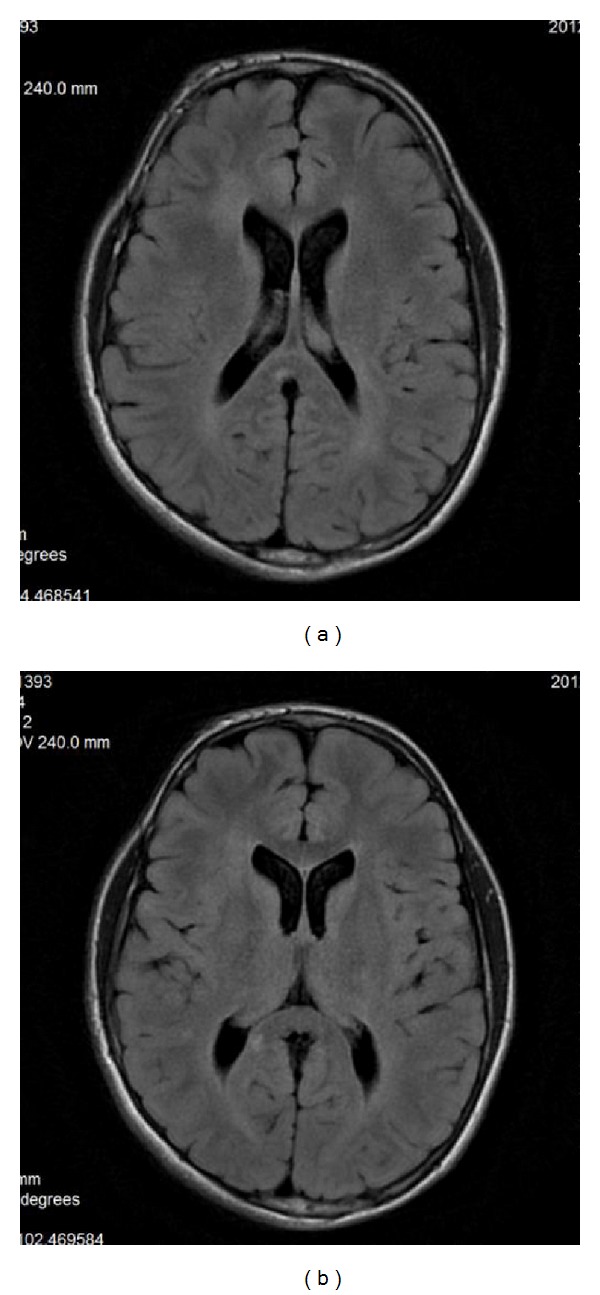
(a), (b) FLAIR sequence, axial plane. 12-year-old boy with NF1. Two hyperintense lesions in the callosal splenium—they were absent on the previous examination at the age of 10.
